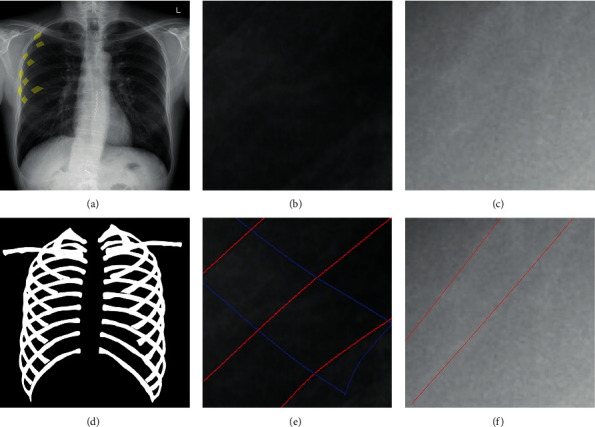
Raw chest X-ray and corresponding marked images. The first column shows the raw chest radiograph and the corresponding mask image. The second column presents a partial enlarged view of the lung area and the corresponding rib border markers. The third column provides a local magnification of the non-lung area and corresponding rib border markers. The edges of the anterior and posterior ribs are marked with blue and red lines, respectively. (a) Raw chest X-ray. (b) Partial enlarged view of the lung area. (c) Local magnification of the non-lung area. (d) Mask image. (e) Rib border markers. (f) Rib border markers.
